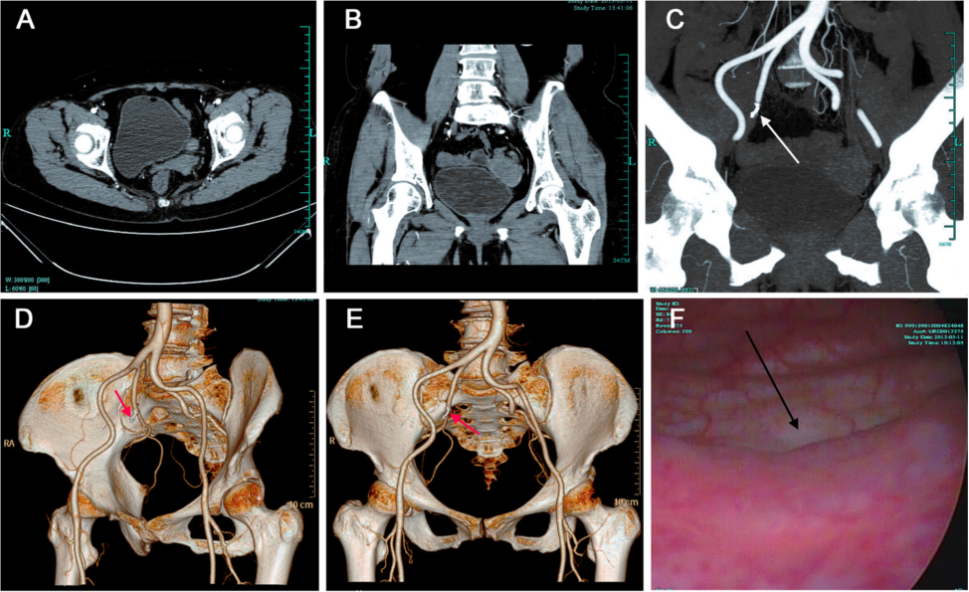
A fellow-up pelvic computed tomography angiography (CTA) 2 months postoperatively showed completely occlusion of superior vesical artery with a coil *(arrow)*. (A) arterial phase CT image; (B) image of coronal CT multiplanar reconstruction. (C) image of coronal CTA multiplanar reconstruction; (D,E) images of three-dimensional CTA. (F) Cystoscopic examination 2 months later revealed a healing mucosa after electrocoagulation *(arrow)* located at the right bladder base adjacent to interureteric fold.
